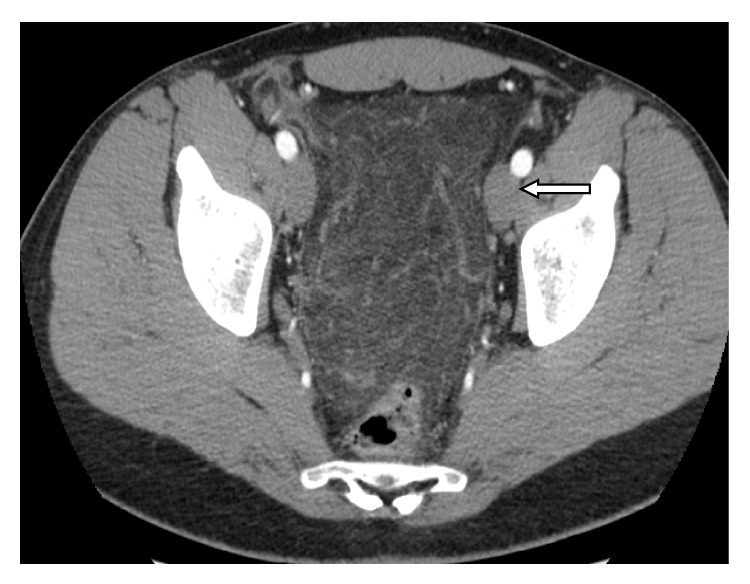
Axial contrast-enhanced CT scans obtained at the pelvis.
